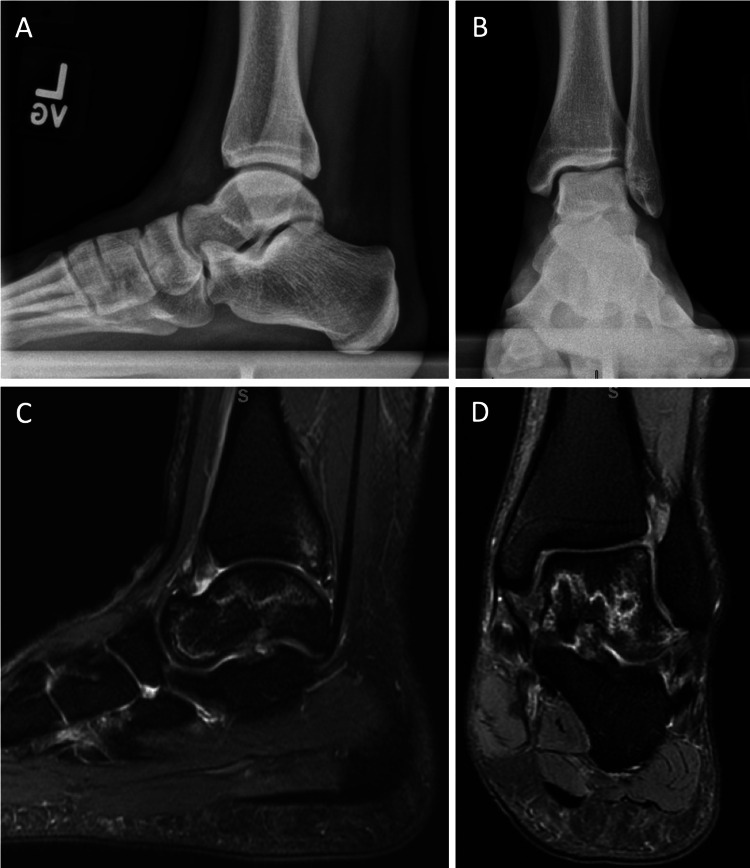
Radiographs and MRI of the left ankle at six months since onset of pain - March 31, 2021. (A-B) Lateral and anteroposterior X-rays demonstrating no acute injury, no signs of peritalar joint degeneration, or sclerosis. (C-D) T2-sequence, sagittal, and coronal views show a hyperintensity line, or “double line sign,” within the ischemic bone marrow and no subchondral collapse. The posterior tibial plafond re-demonstrates a small osteochondral defect, unchanged from the prior exam.
